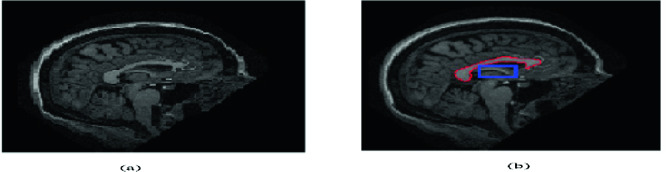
Example of sagittal brain MRI slices from the OASIS dataset: (a) The input MRI. (b) Delineation of the CC, where the fornix (framed in blue) appears in the neighborhood of the CC while being of similar appearance. (Color figure online)
